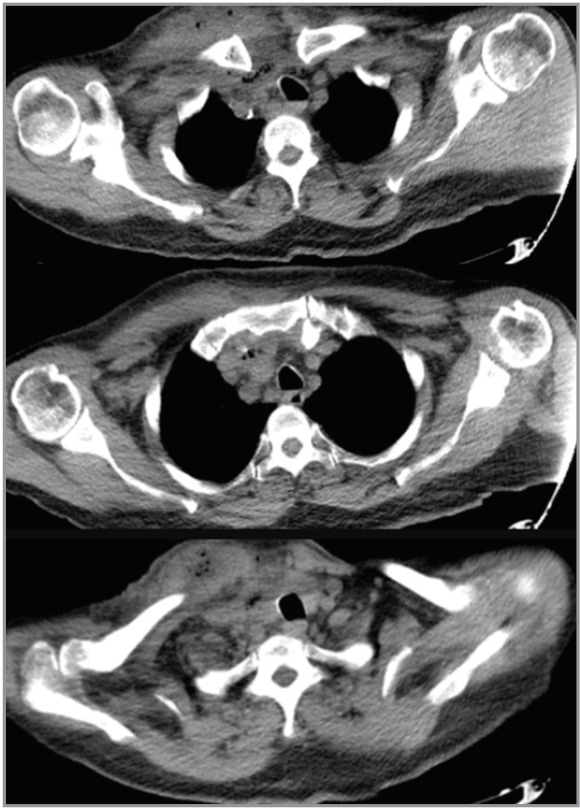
Mediastinal section of a thoracic computed tomography
